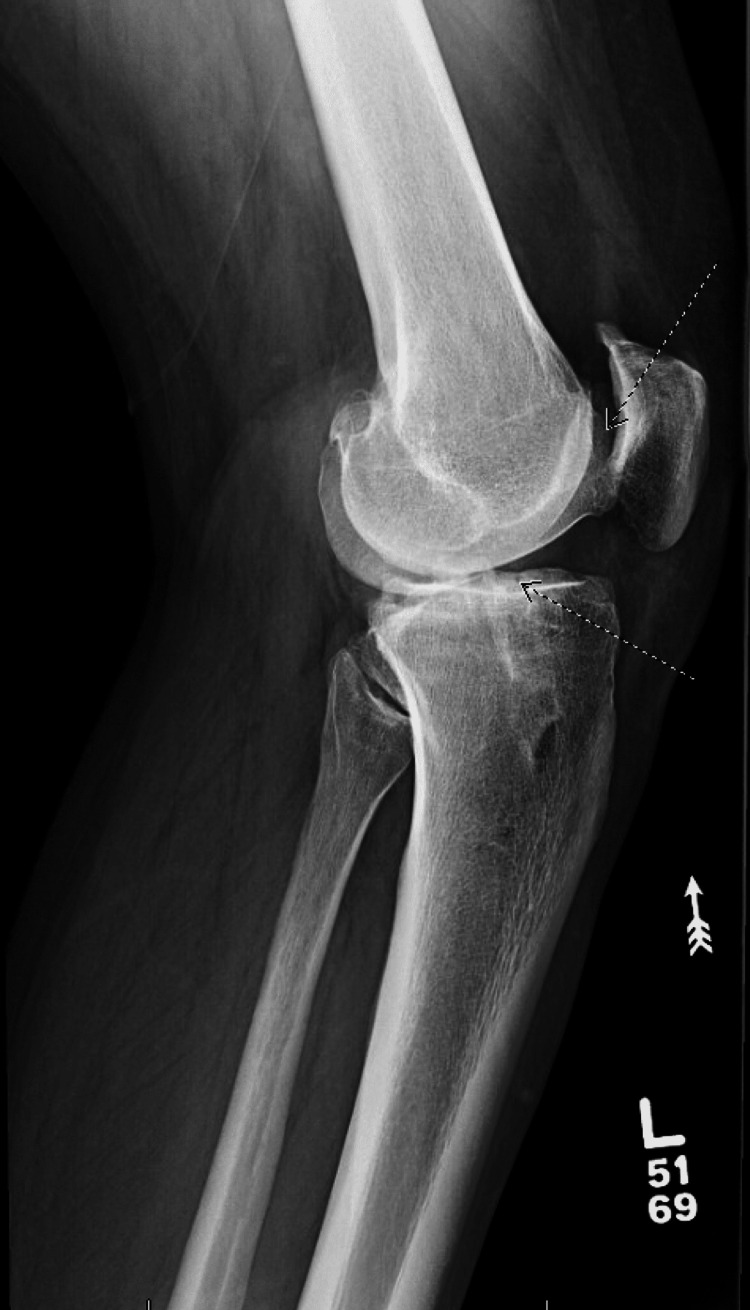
Preoperative radiographs. Lateral view of left knee, showing degenerative changes. Dotted arrows show diffuse joint space narrowing and osteophytes.
